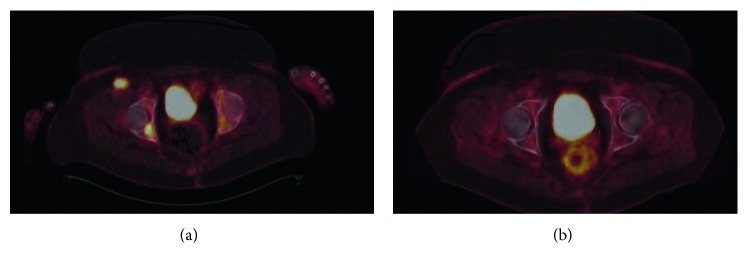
Fused PET-CT images: bone window showing resolution of bone lesions (a) prior to lenalidomide use and (b) after lenalidomide use.
